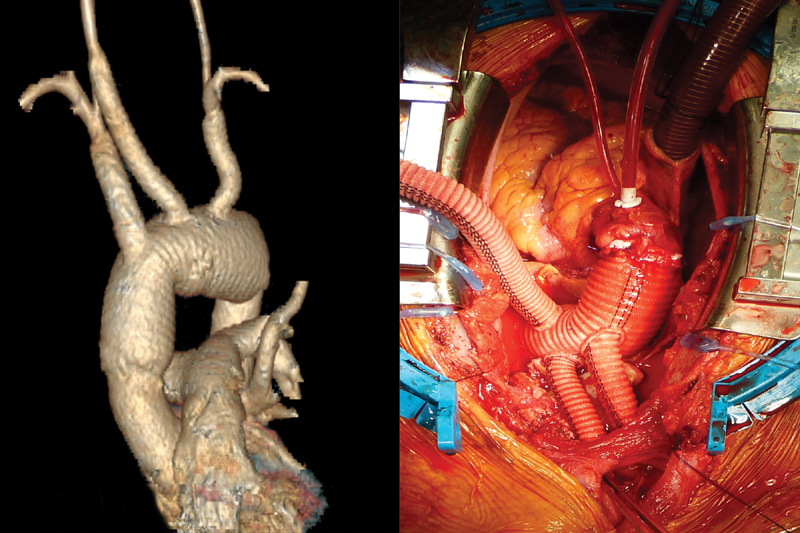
Postoperative computed tomography scan reconstruction (left). Operative view (right).
